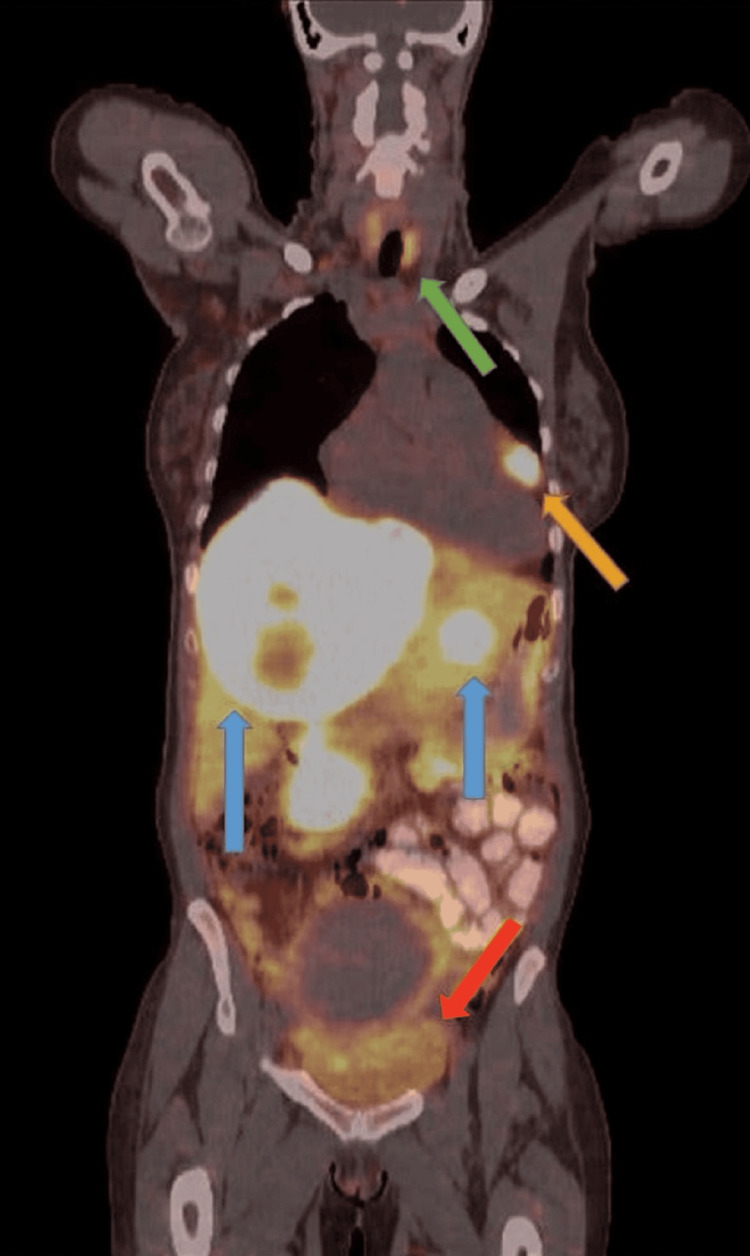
PET DOTATATE There were multiple intensely DOTATATE avid bilobar hepatic masses, including dominant segment 4/8 centrally photopenic/necrotic mass, corresponding to the biopsy-proven neuroendocrine tumor (blue arrow). Also, there was an intensely DOTATATE avid left anterior pericardial soft tissue mass inseparable from the right ventricle, probably nodal versus metastatic involvement (orange arrow). There were additional low-level DOTATATE avid prominent bilateral supraclavicular, mediastinal, and abdominopelvic lymph nodes, with uptake below the background level, possibly reactive versus disease-related (green arrow). There was enlarged leiomyomatous uterus with heterogeneous DOTATATE avidity. Pelvic ultrasound was consistent with a large myoma measuring up to 8 cm (red arrow).
